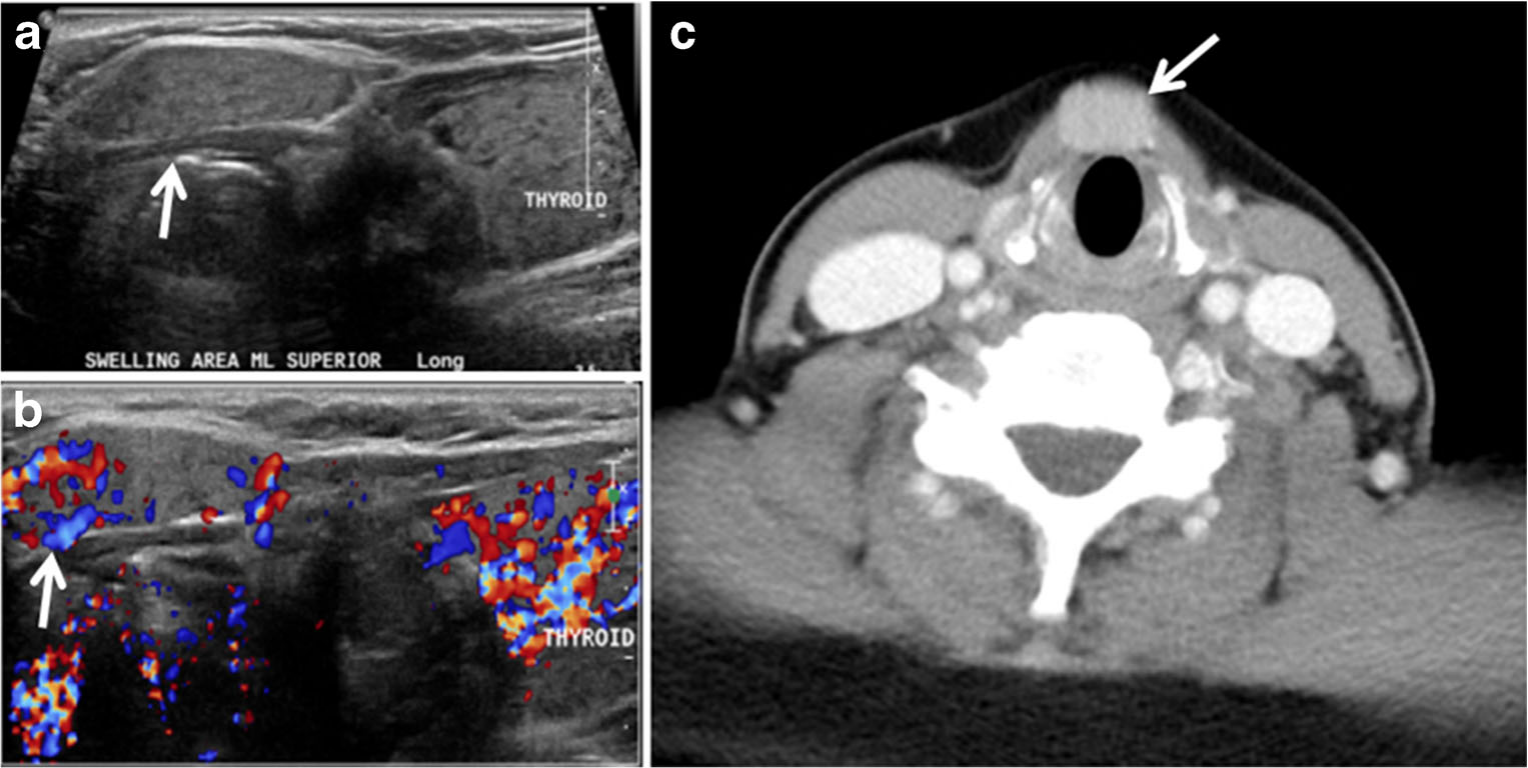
Midline ectopic thyroid with Hashimoto’s thyroiditis in a 49-year-old female patient. a Transverse greyscale ultrasound shows a 1.6 × 0.8 cm solid, well-defined, heterogeneous area (*white arrow*) in the midline, superior to the thyroid gland. It is iso-echogenic to the thyroid gland with no definite connection to the thyroid gland. b Transverse colour Doppler ultrasound shows significant increase in vascularity. c Axial enhanced neck CT scan at the level of thyroid cartilage demonstrates midline infrahyoid hyperdense soft tissue mass (*white arrow*) embedded within the strap muscle
